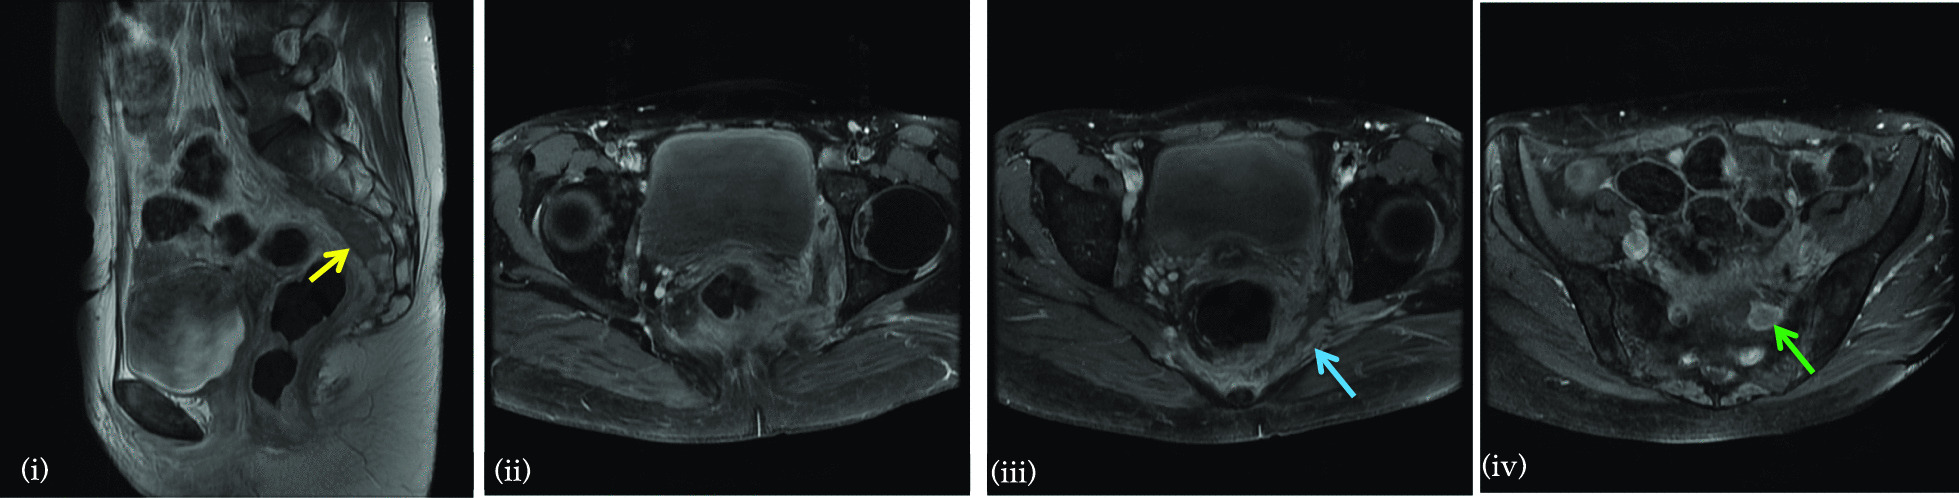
Magnetic resonance imaging of the pelvis with contrast performed for further evaluation of the soft tissue abnormality seen on computed tomography (Fig. 1). (i) Sagittal T2WI demonstrates soft tissue thickening in the presacral space (yellow arrow). Post-contrast fat-saturated axial T1WI ((ii)) and (iii) demonstrate enhancing soft tissue in the left pelvic side wall, left mesorectal fat, and extending through the greater sciatic foramen (blue arrow). (iv) Soft tissue could be seen to extend toward the left L5/S1 exiting neural foramen with involvement and thickening of the exiting left-sided sacral nerve roots (green arrow), lumbosacral plexus and ipsilateral sciatic nerve
